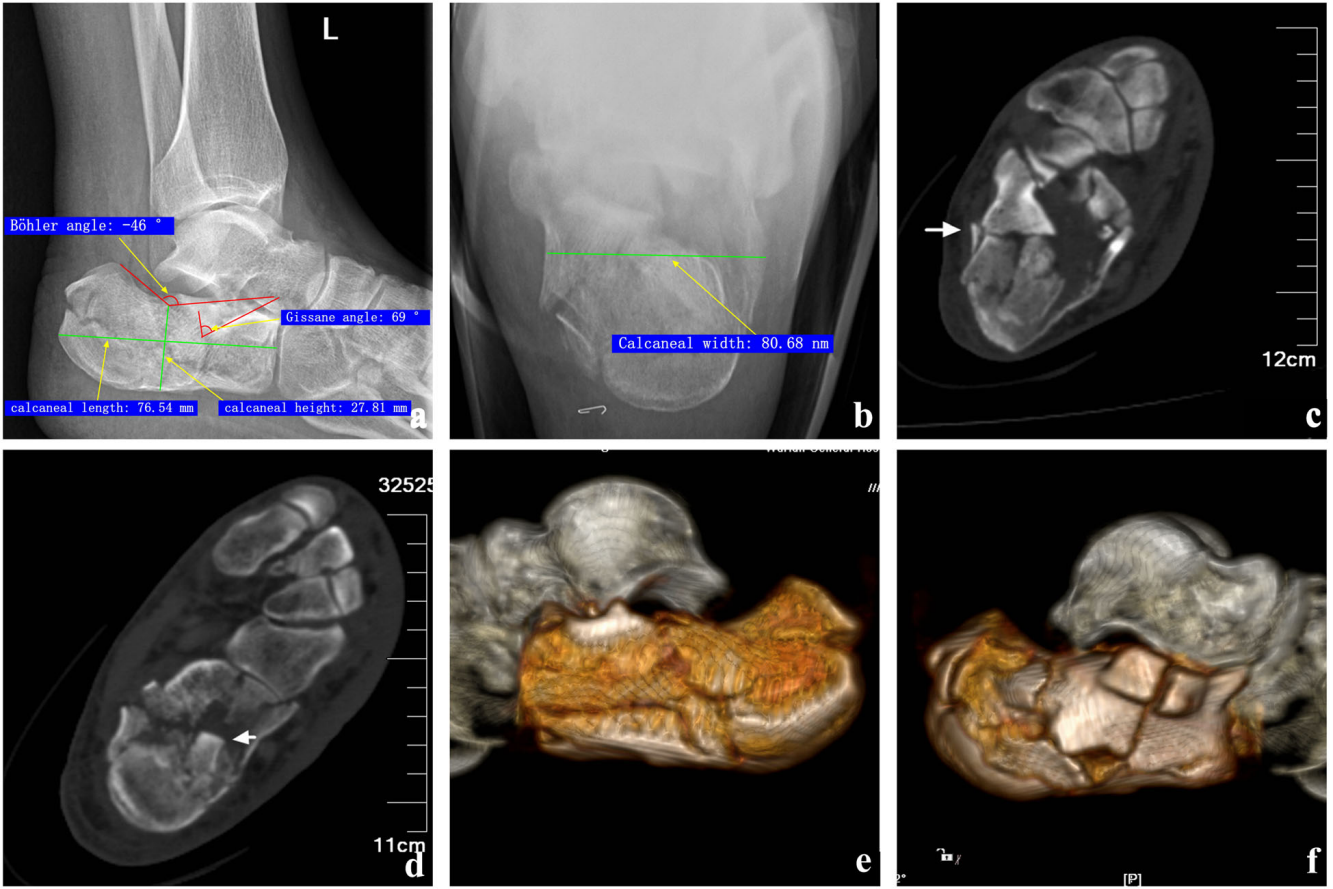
Sanders type III calcaneal fracture in a 47-year-old male. a Preoperative lateral axial radiograph of the calcaneus shows articular surface collapse. Böhler angle, − 46°; Gissane angle, 69°; calcaneal height, 27.81 mm; calcaneal length, 76.54 mm. b Preoperatively, the calcaneus is widened. Calcaneal width, 80.68 nm. c, d The articular surface has a comminuted fracture, and the middle mass of the bone is sunk into the calcaneus. e, f CT demonstrated calcaneal medial wall crush, and intercalary displacement of bone mass
